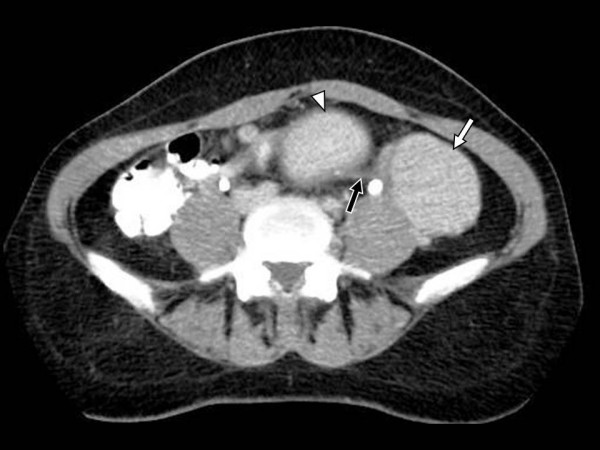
Thin section contrast-enhanced computed tomography of the lower abdomen. Thin section contrast-enhanced CT scan targeted at the level of the mass reveals a thin stalk (black arrow) connecting the mass (white arrow) with the upper left body of the uterus (arrowhead).
